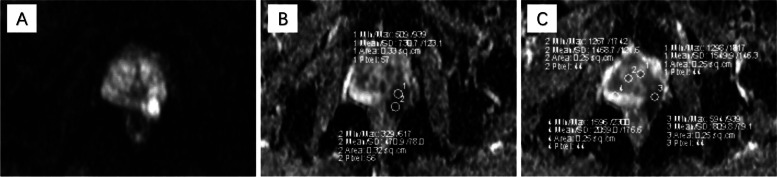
ADC measurements of prostate cancer. A shows an abnormally high signal focus in the left peripheral zone on the DWI (B-value 1000 mm/s^2^); B, C show that the mean ADC value of the lesion in the left peripheral zone is 0.670 × 10^-3^mm^2^/s
